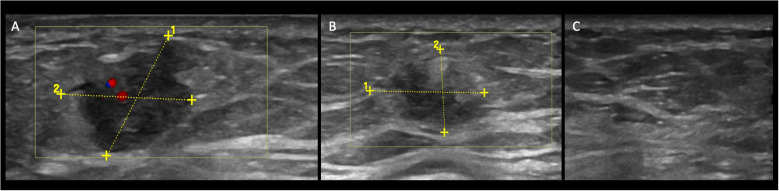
Case patient no. 4. (A) Color-Doppler US demonstrates a hypoechoic mass with an irregular shape and not-circumscribed margin, vertical orientation posterior, and internal vascularization at baseline. (B) At T1, the lesion changed the size (14 mm to 11 mm), and the vascularization at Color Doppler is now absent. The echo pattern remained hypoechoic. At T2 (C), the lesion is not visible anymore.
